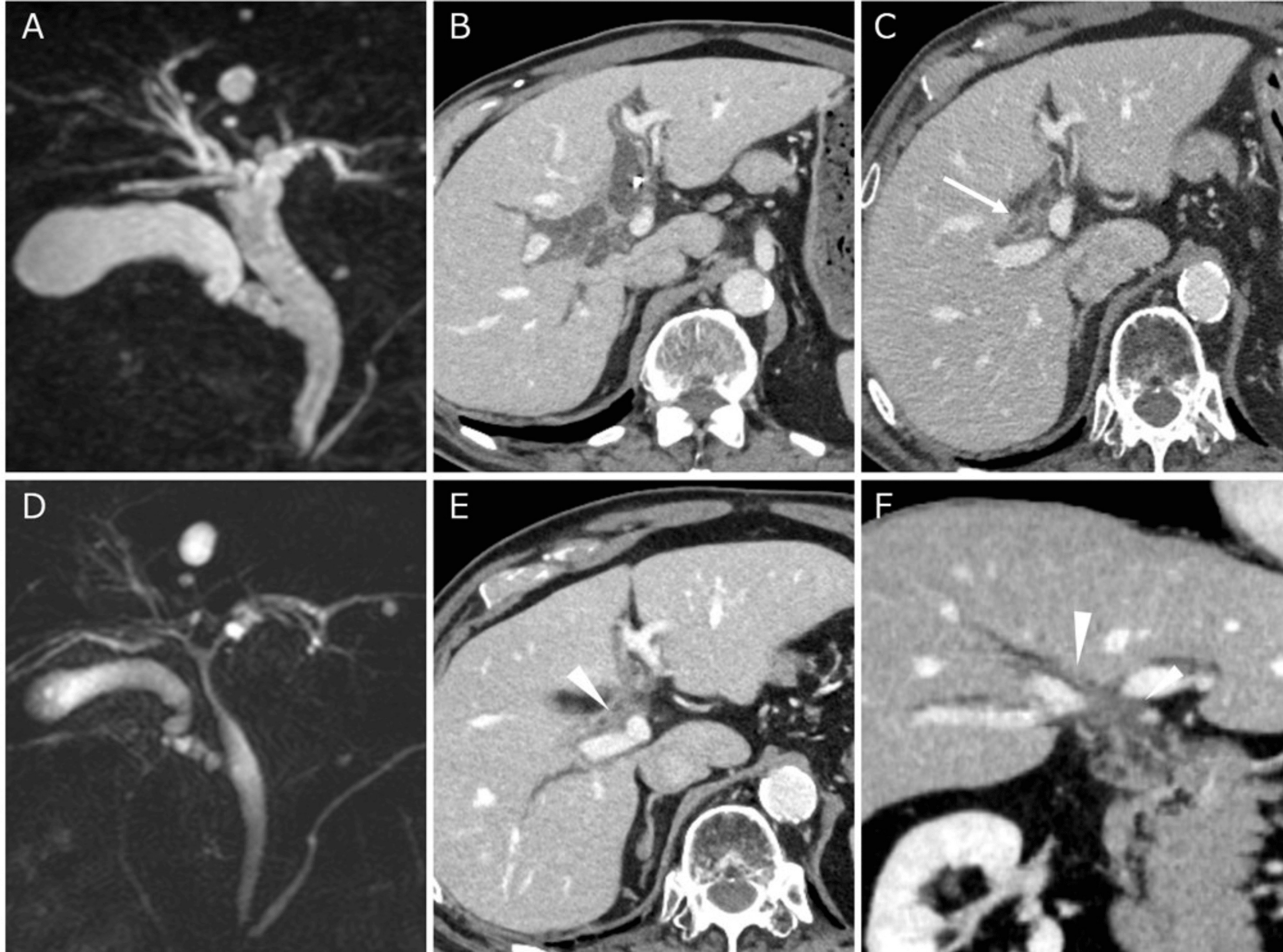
Comparison of MRCP and enhanced CT pre- and post-stereotactic body radiotherapy MRCP: magnetic resonance cholangiopancreatography; CT: computed tomography The biliary duct dilation observed in the pre-treatment MRCP (A) and the contrast-enhanced CT axial image (B). Enhanced CT at the end of radiation therapy showed improvement in biliary duct dilation (arrow). (C). No biliary duct dilation was observed in the MRCP performed at nine months (D) and in the CT scan after one year post-irradiation (arrow head) (E, F).
